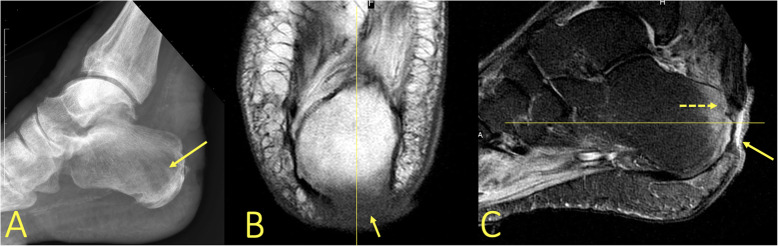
A 66-year-old male with diabetes. a Osteomyelitis in calcaneus was suspected clinically and on radiographs. b, c MRI confirmed the diagnosis (arrows), BME (dashed arrow)
